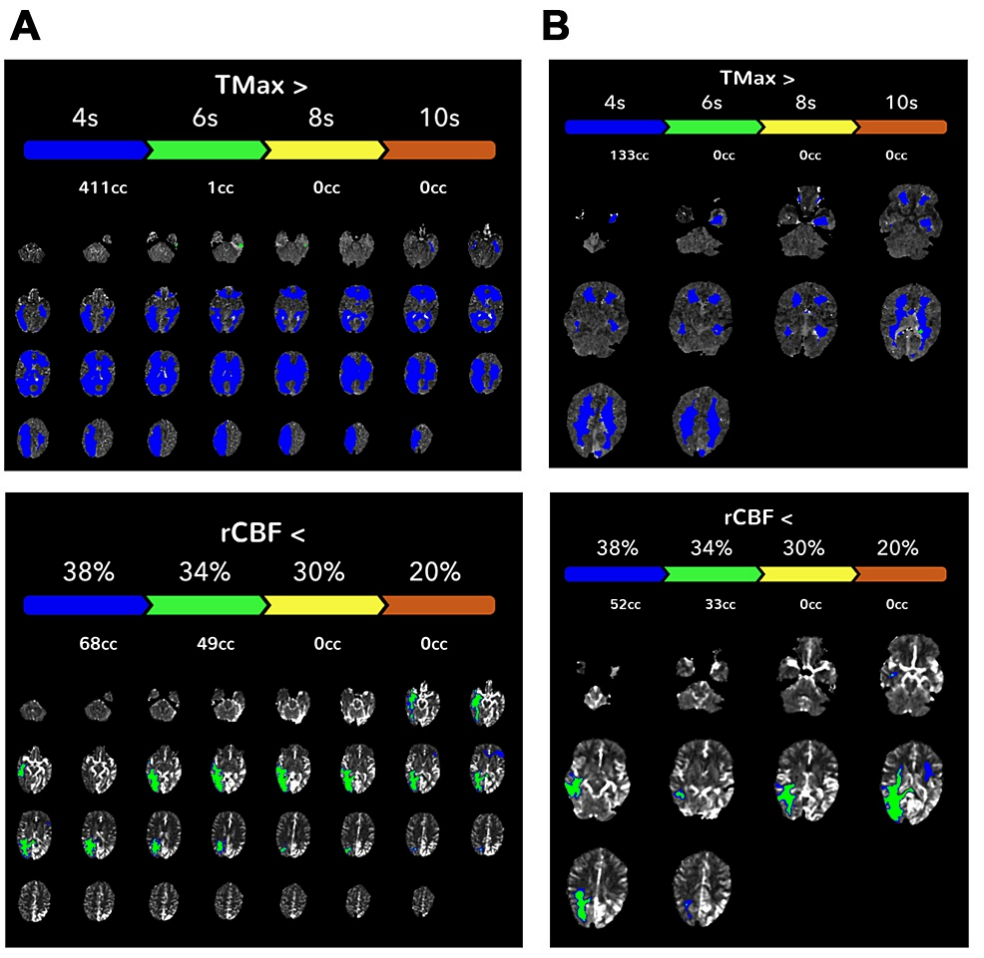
CT perfusion imaging at initial imaging. (A) Pre-acetazolamide studies reveal diffusely increased time-to-maximum (Tmax; >4 seconds) in bilateral cerebral hemispheres and decreased regional cerebral blood flow (rCBF) in the left parietal and temporal lobes. (B) Upon acetazolamide administration, there was an improvement in the hemodynamics metrics, with a decreased extent of prolonged Tmax.
